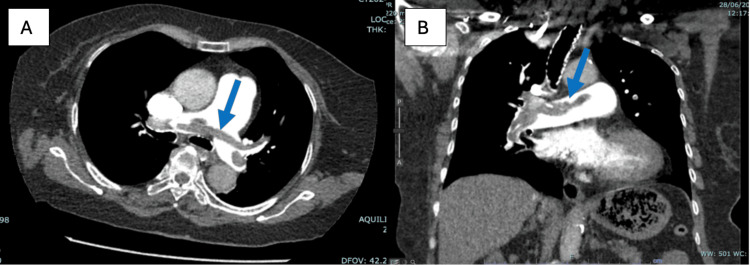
CT pulmonary angiogram (A: axial view and B: coronal view) showed extensive saddle embolism at the bifurcation of the pulmonary trunk (the arrow indicates the pulmonary thrombus).
